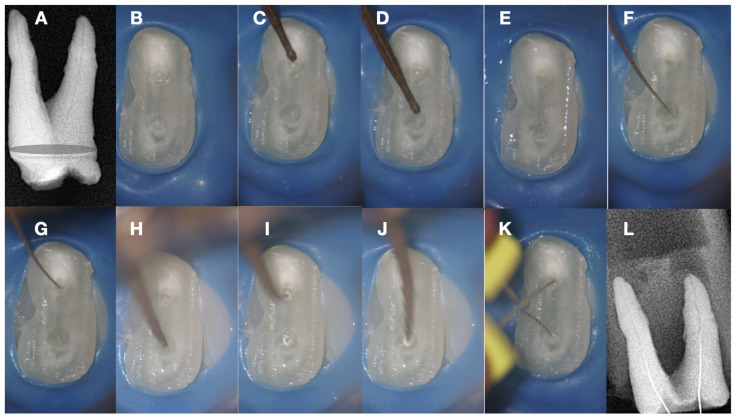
(A) Radiographic image of an extracted partially calcified maxillary premolar. (B) Magnified view at the level of the axial cut (a grey area in a). (C,D) Creation of initial access dimple in the white spot indicating the calcified orifice. (E) Continuous chelation of the cut dentinal surface (F,G). An initial unsuccessful attempt to negotiate the calcified canal orifices with D-finder files iso 08. (H) Fitting of the tip of an EDM file (Hyflex EDM-one file, Coltene) in the dimple without activation. (I,J) On-spot buckling resistance activation test (BRAT) negotiation of the EDM files. (K) Negotiation of the 08 d-finders inside the calcified canals after the BRAT negotiation technique removed coronal calcified canal obstructions. (L) Radiographic verification of calcified canal negotiation (images and radiographs courtesy of Dr. Chaniotis Antonis).
